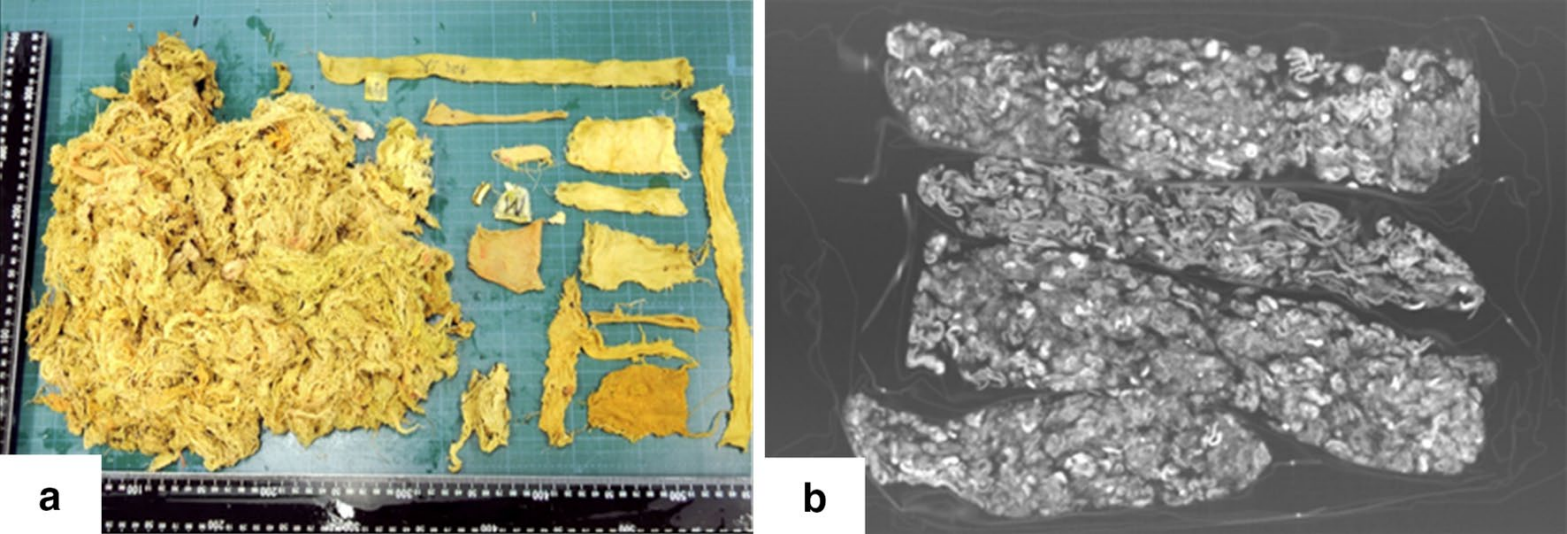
Image of gastrointestinal tract materials. a The gastrointestinal tract was found to contain copious amounts of cloth pieces (aggregate amount: 738 g). b A piece of cloth located in the intestinal tract was scanned by using computed tomography. However, this was not similar to the imaging findings of postmortem computed tomography.
